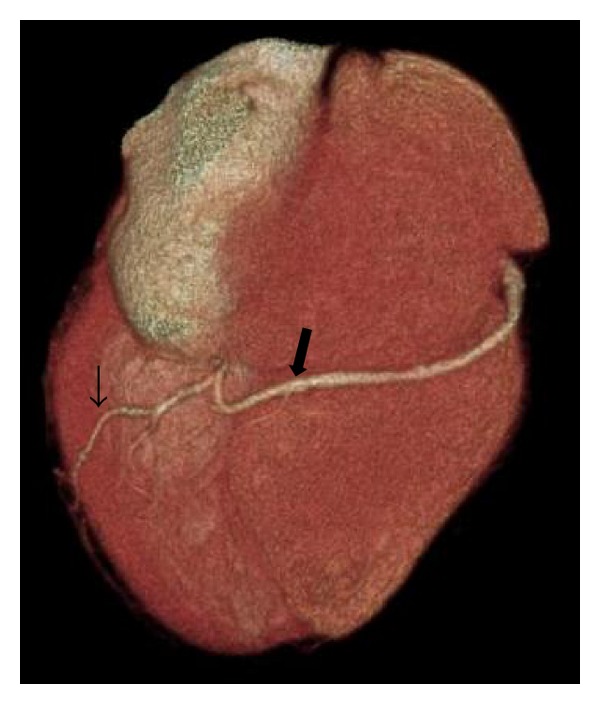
Inferior view of three-dimensional computed tomography scan shows superdominant right coronary artery (large arrow) to supply posterior wall (small arrow).
